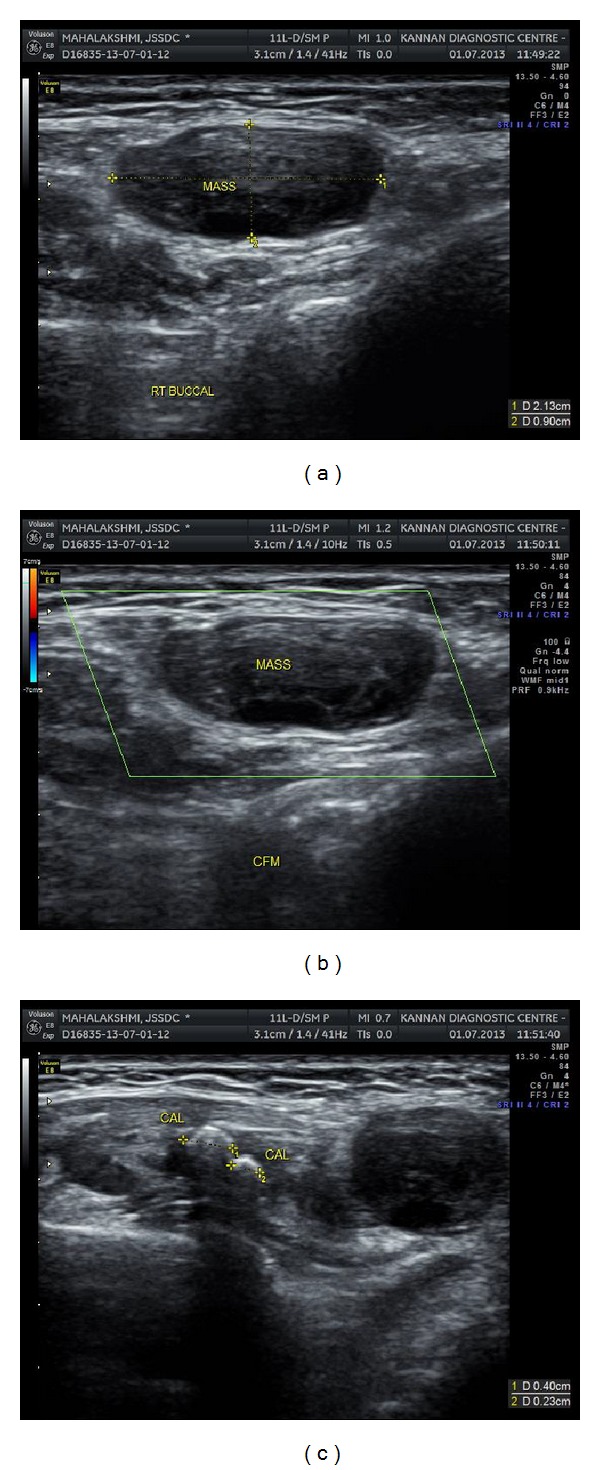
(a) Ultrasonography revealed a well-defined cystic mass within the masseter muscle. (b) Color doppler sonography indicated the mass to be avascular. (c) Ultrasonographic appearance of the calcific masses.
